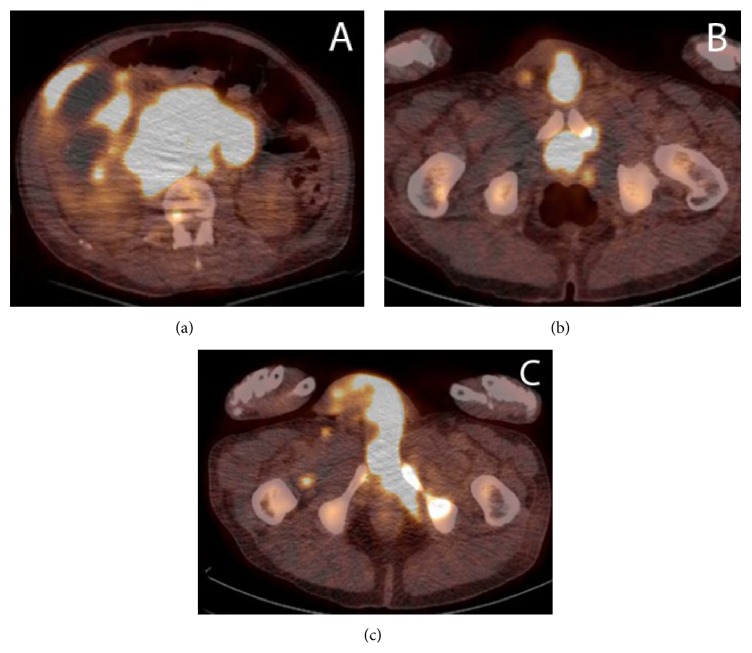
(a) Evidence of intense FDG uptake in the right kidney as well as retroperitoneal lymph nodes. (b) Intense FDG uptake in the prostate with extension to the base of the penis. (c) Intense FDG uptake in the base of the penis with minimal extension into the shaft.
